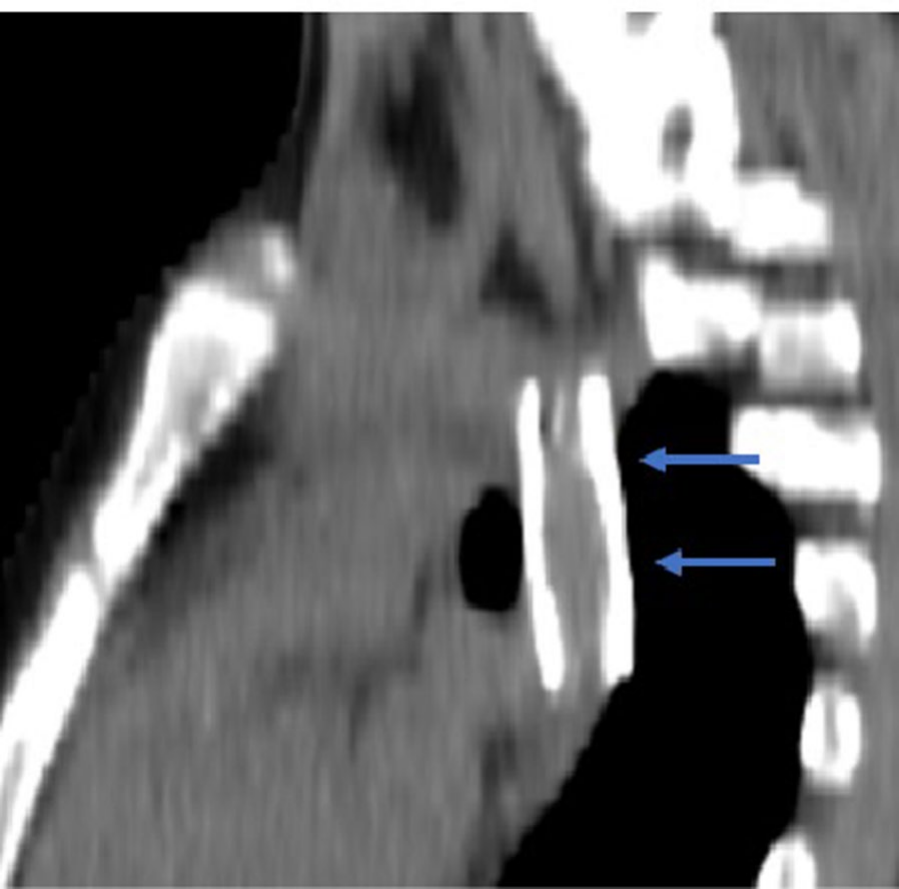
Follow-up CT image at 6 months showing well-expanded stent with no complications
